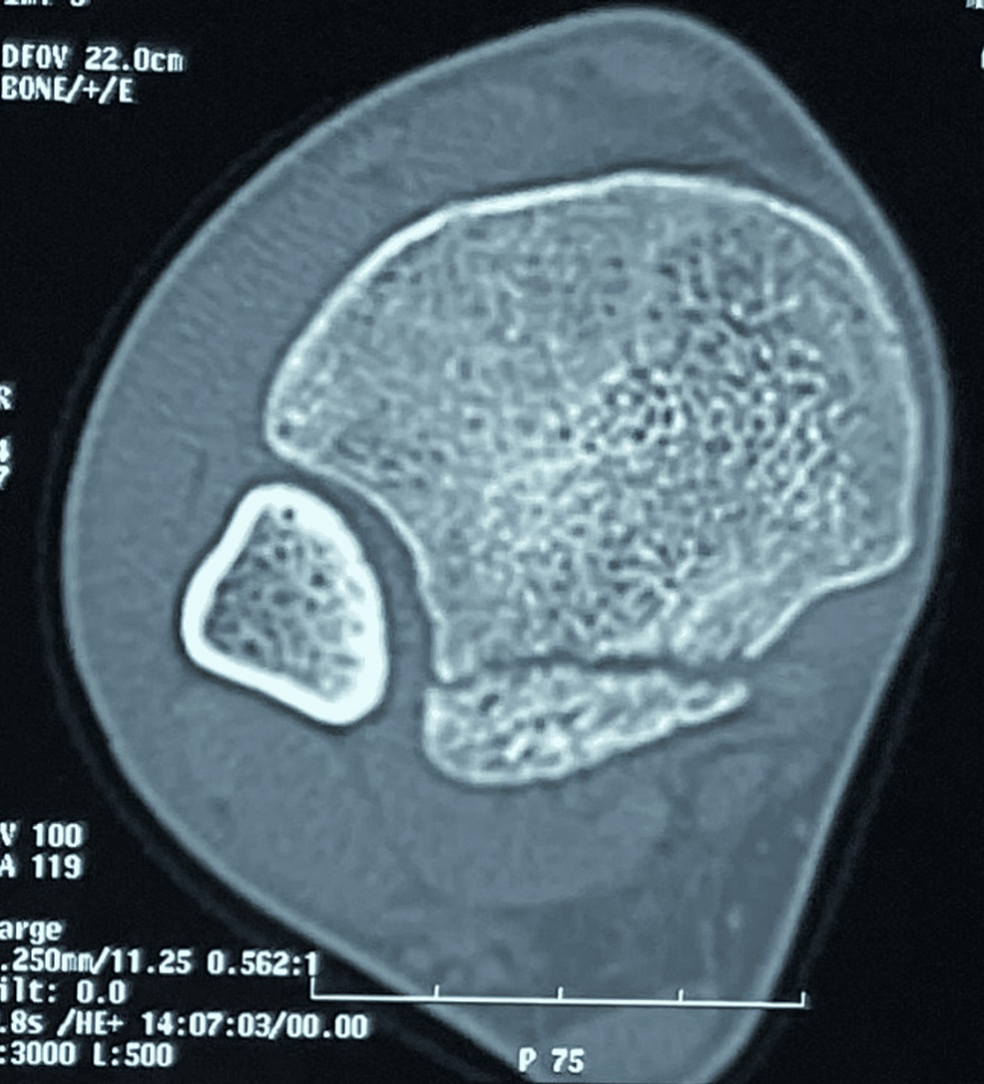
A transverse section of the non-enhanced CT scan of the ankle confirming the isolated fracture of the posterior margin
